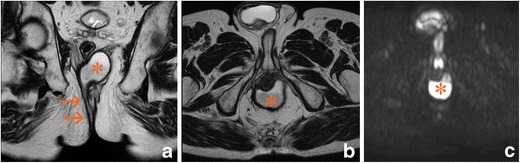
Right suprasphicteric fistula (arrows) with retroanal abscess (asterisk). a Oblique-coronal T2-weighted image. b Oblique-transverse T2-weighted image. c Native oblique-transverse native diffusion-weighted image with 800 s/mm^2^ b factor
